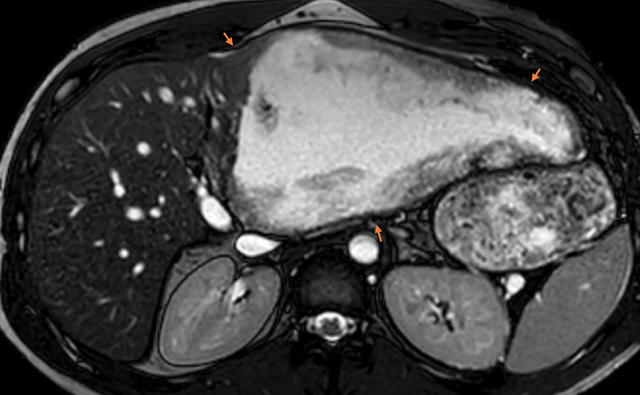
Axial T2 TSE MRI. The left hepatic mass (orange arrows) has a necrotic content (hyperT2) with peripheric fibrosis (hypoT2) and microcyst (hyperT2).
